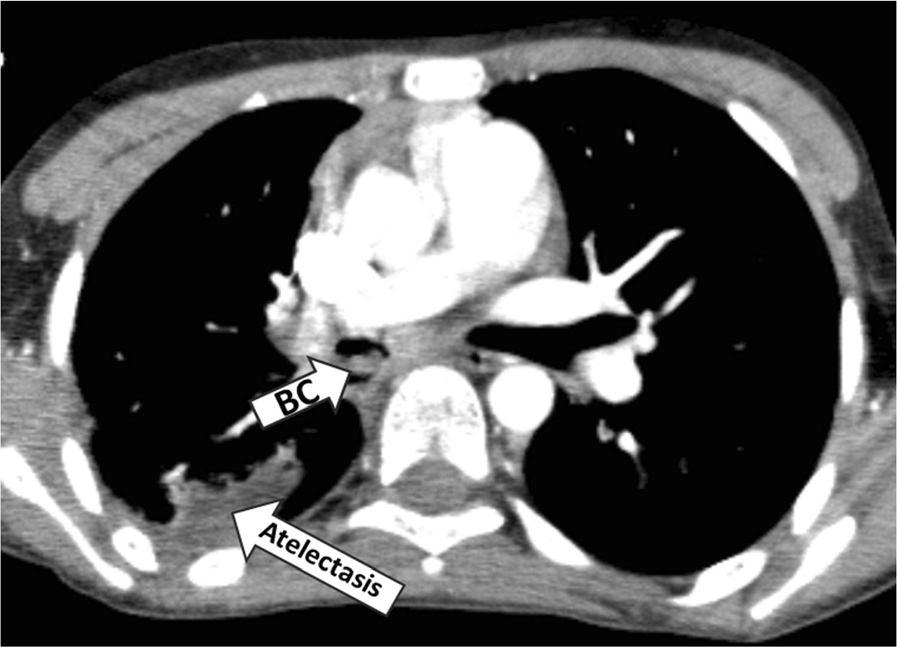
Thoracic computed tomography of case 1, revealing a solid 18 × 5 mm mass in the right main bronchus (BC) and post-obstructive atelectasis in the right upper lobe
